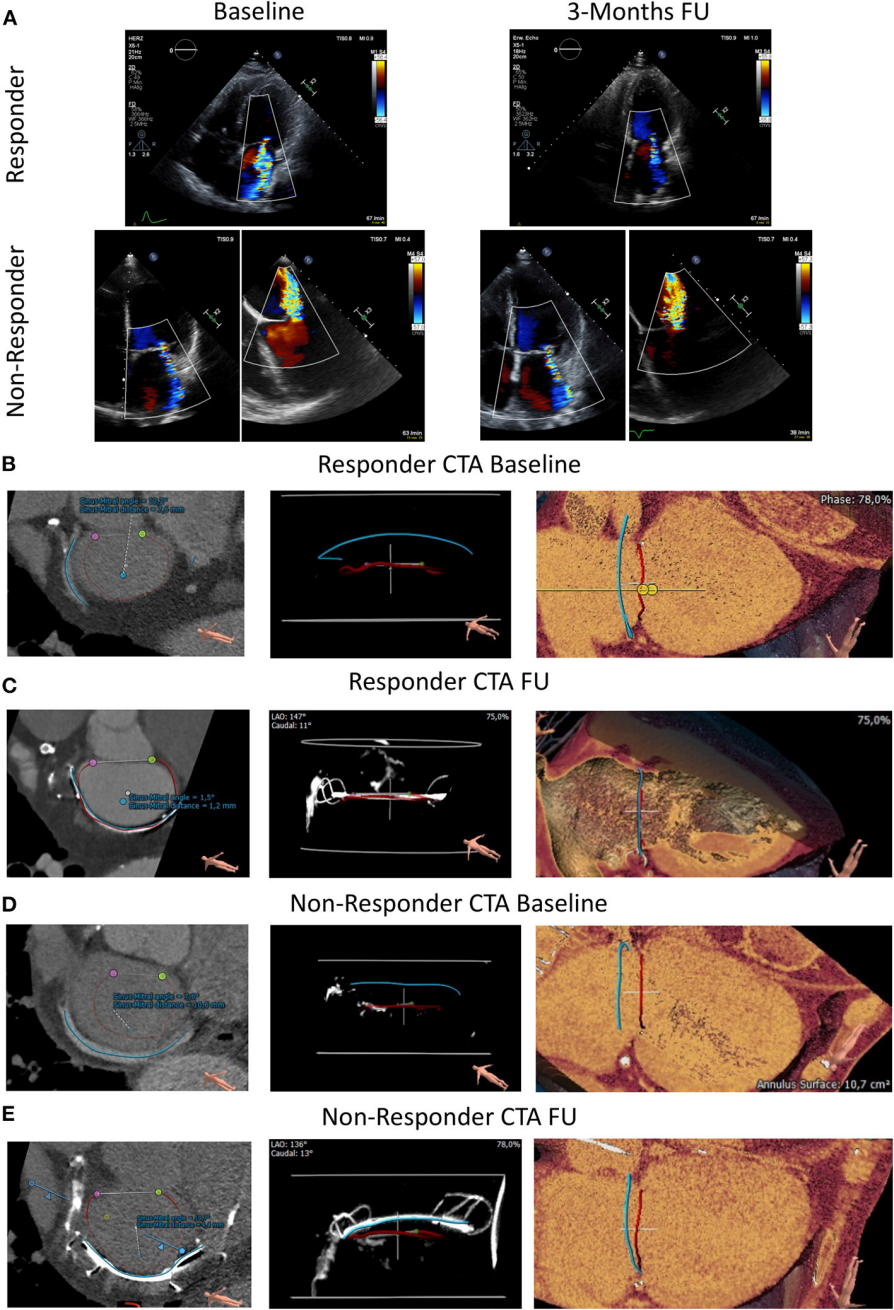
Transthoracic echocardiography and CTA at baseline and 3 months follow-up in an FMR responder and non-responder following Carillon device implantation. (A) Transthoracic echocardiographic at baseline and 3 months FU (follow-up) in an FMR responder following indirect mitral valve annuloplasty. Transthoracic and transesophageal echocardiography at baseline and 3 Months FU (follow-up) in an FMR non-responder following Carillon device implantation. (B, D) CTA prior index procedure at baseline of a responder (B) and non-responder (D). Blue Line: Coronary Sinus; Red Line: Mitral Valve Annulus; Purple and Green Dot: lateral and medial trigone. Distance and angulation of CS to MVA plane as indicated. (C, E) CTA post device implantation at follow-up of a responder (C) and non-responder (E). Blue Line: inner curvature of the Carillon device; Red Line: Mitral Valve Annulus; Purple and Green Dot: lateral and medial trigone. Distance and angulation of the Carillon device to MVA plane reflects the sinus mitral angle and distance (as indicated).
